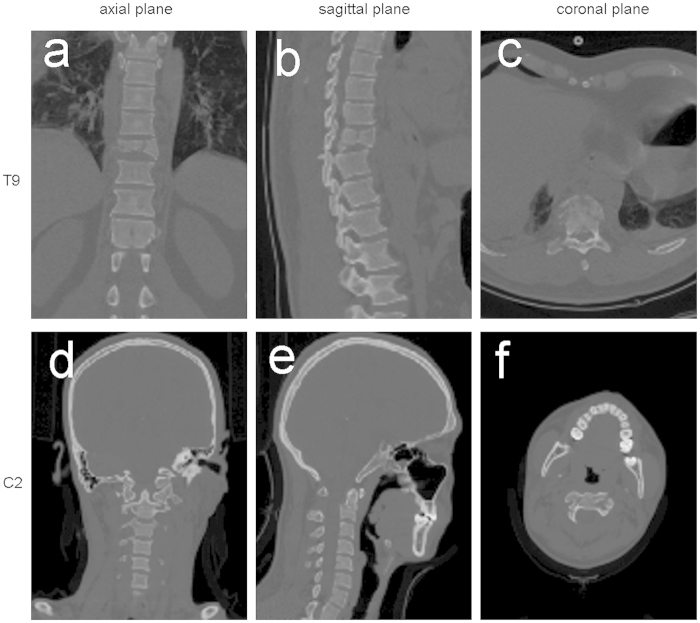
Screenshot of the teaching module for two-dimensional computed tomography images. The ninth thoracic vertebra (T9):a, b, and c (axial, sagittal, coronal views, respectively); the second cervical vertebra (C2): d, e, and f (axial, sagittal, coronal views, respectively).
